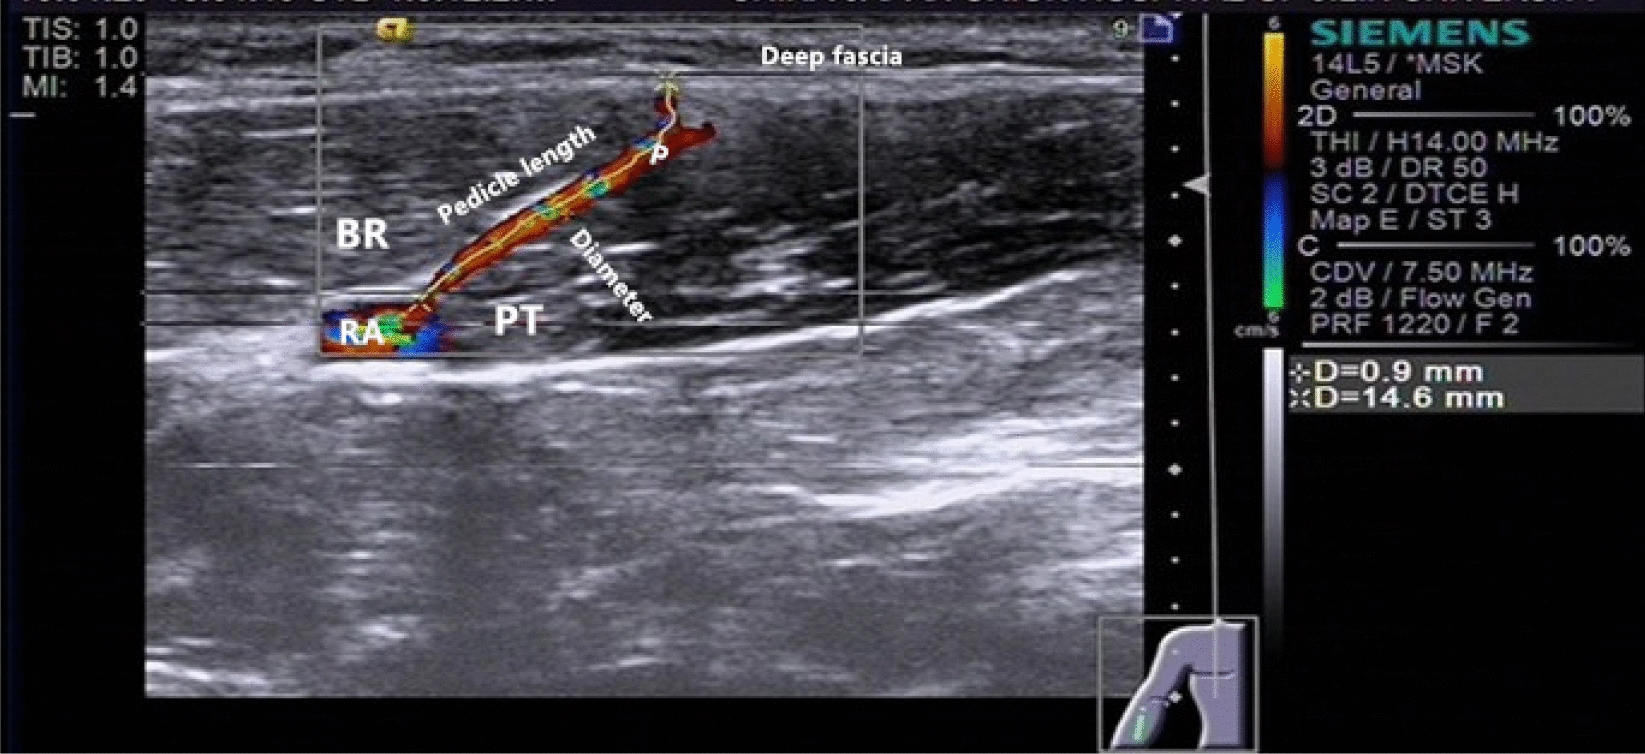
Perforator of Duplex ultrasound image: a perforator arising from the radial artery between the brachioradialis muscle and pronatorteres muscle in the proximal half of forearm. Diameter(+): the internal diameter of the perforator; Pedicle length(×): the pedicle distance from its origination of radial artery to deep fascia; *RA* radial artery; *P *perforator; *BR* brachioradialis muscle; *PT* pronatorteres muscle
